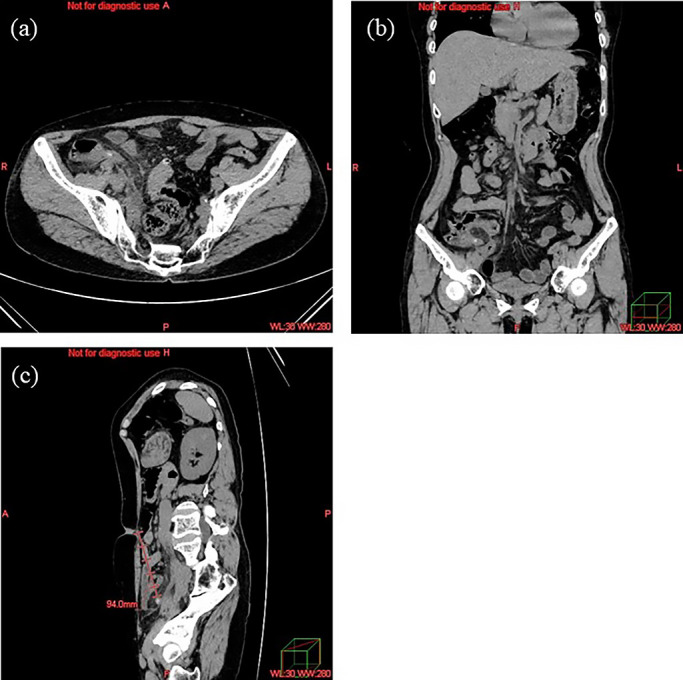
Computed tomography (CT) images at the level of the umbilicus. (a) The appendix is
